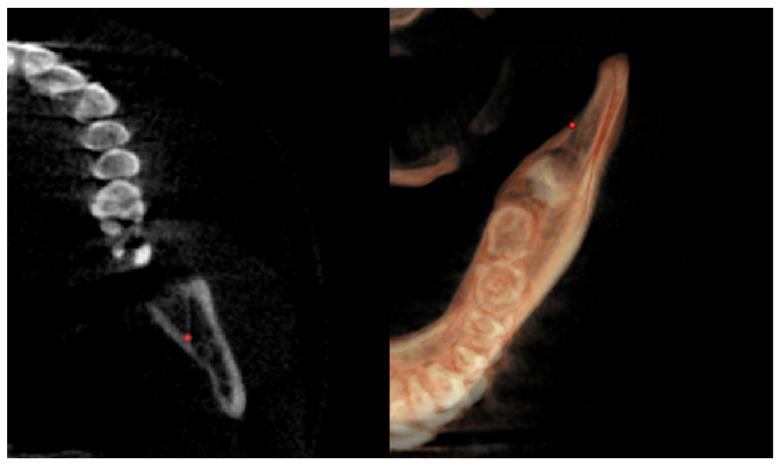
CBCT images showing the anatomical lingual tuberosity.
